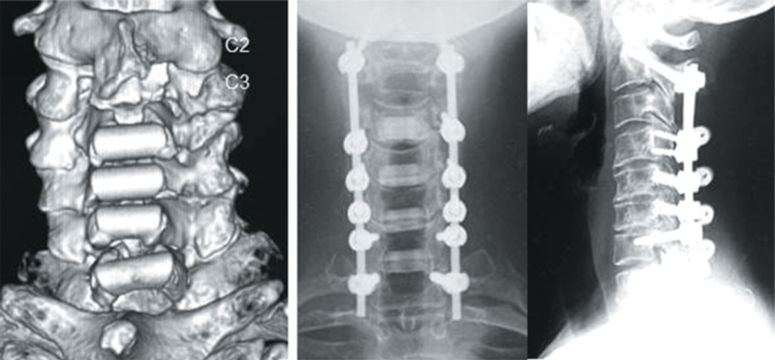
Double-door laminoplasty using hydroxyapatite spinous process spacers at C4-C7 with C3 laminectomy preserving all muscle insertions at C2, and C2-T1 posterior decompression and fusion. Left: Anteroposterior three-dimensional computed tomographic image of cervical laminoplasty alone (C3 laminectomy and C4-7 laminoplasty). Middle: anteroposterior and right: lateral radiographs of the C2-T1 posterior decompression and fusion included C3 laminectomy, C4-7 laminoplasty, and C2-T1 instrumented fusion.
